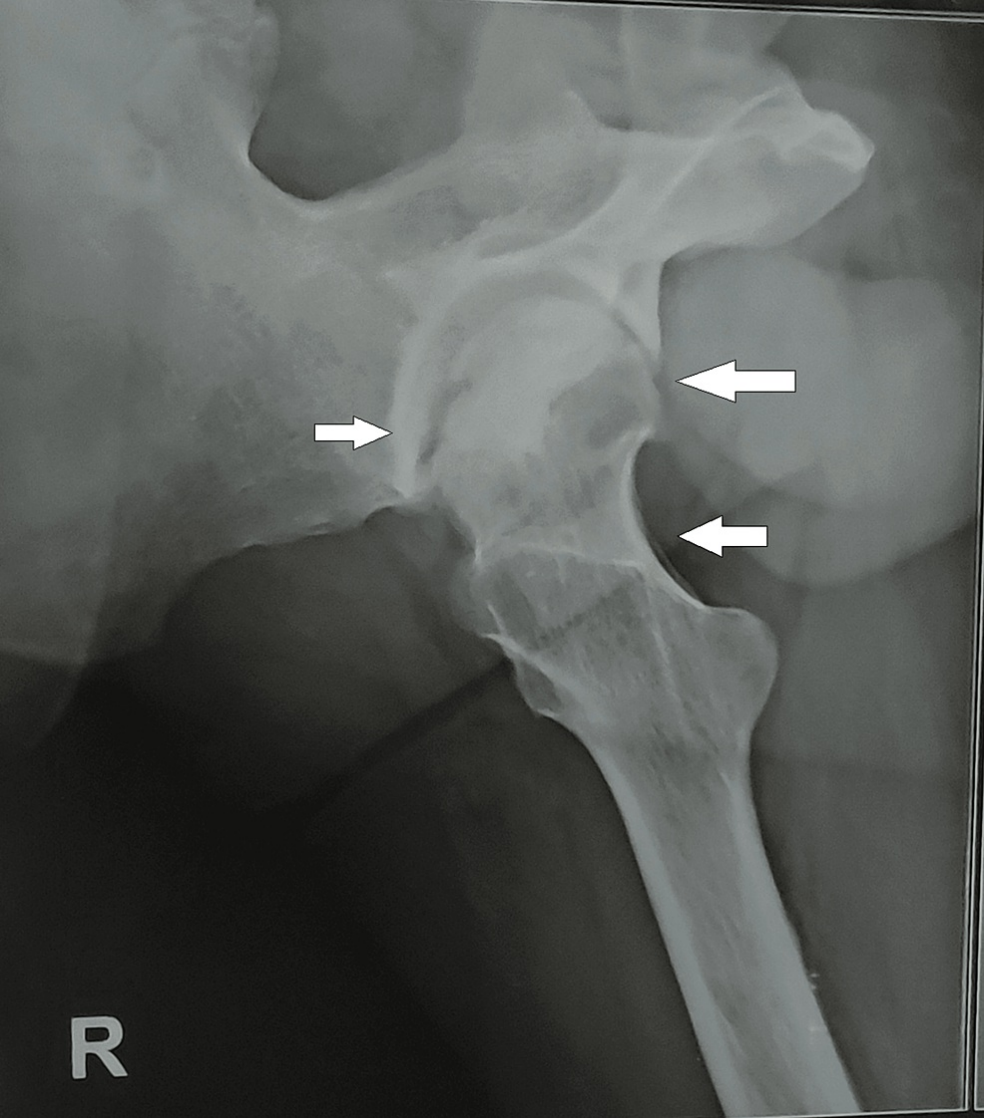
Lateral view of an X-ray showing the destruction of the femoral head on the right side.
